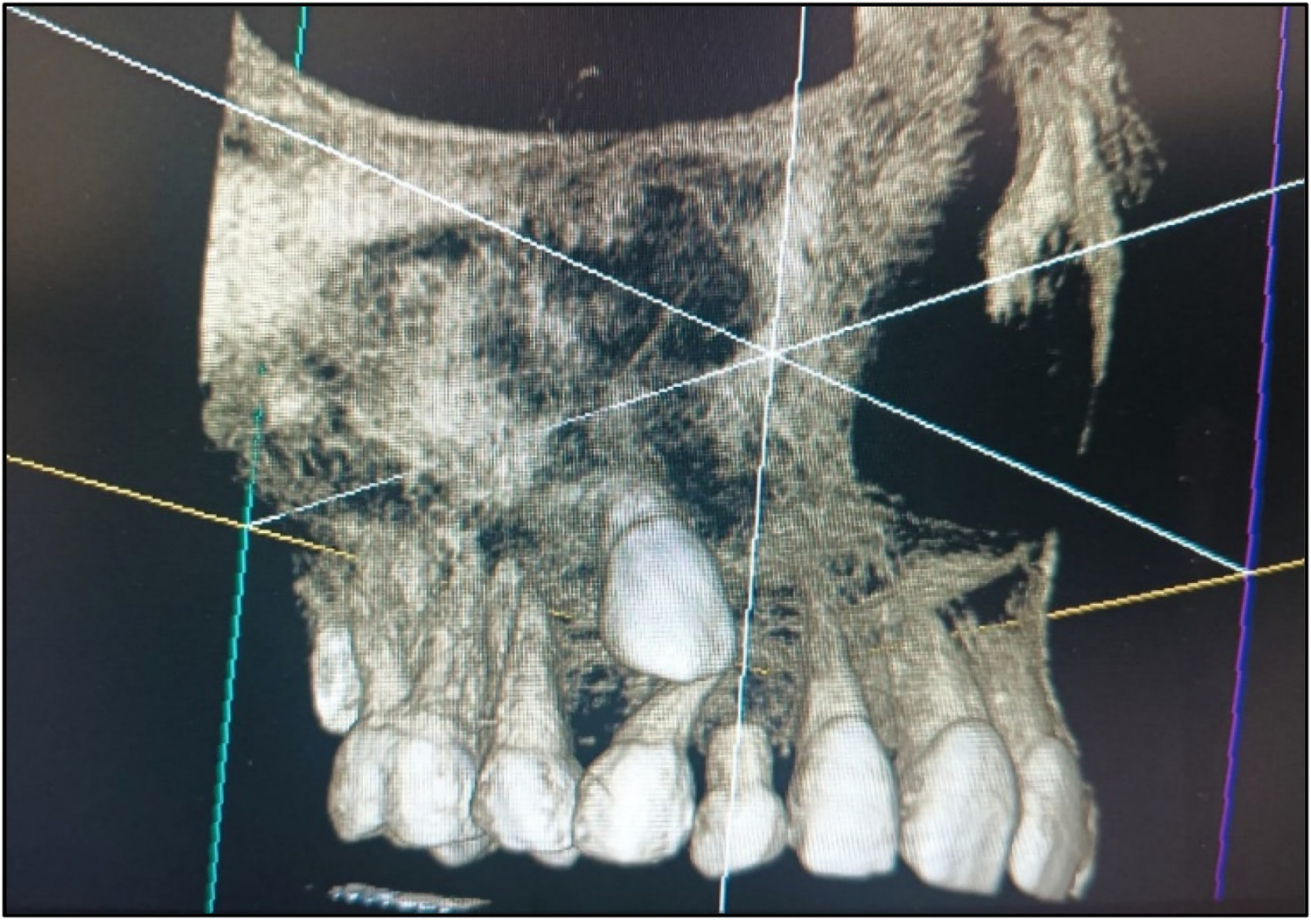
CBCT w.r.t. the 13, 14, 15 region.
